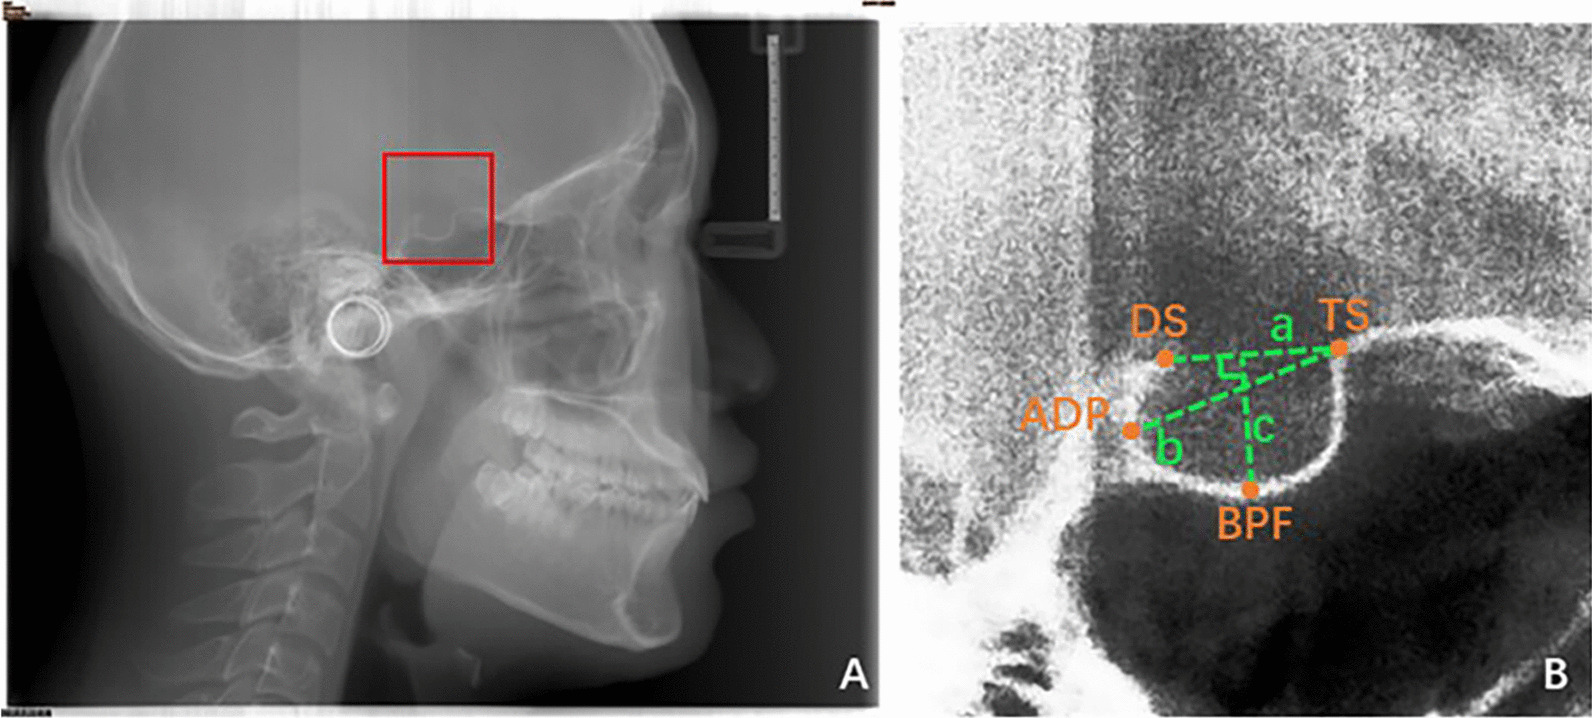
Dimensions of Sella Turcica. A An original X-ray image. The structure in the red box is the ST. B The region of the ST. TS, DS, ADP, and BPF were the four landmarks of ST, line a is Length, line b is Diameter, and line c is Depth
